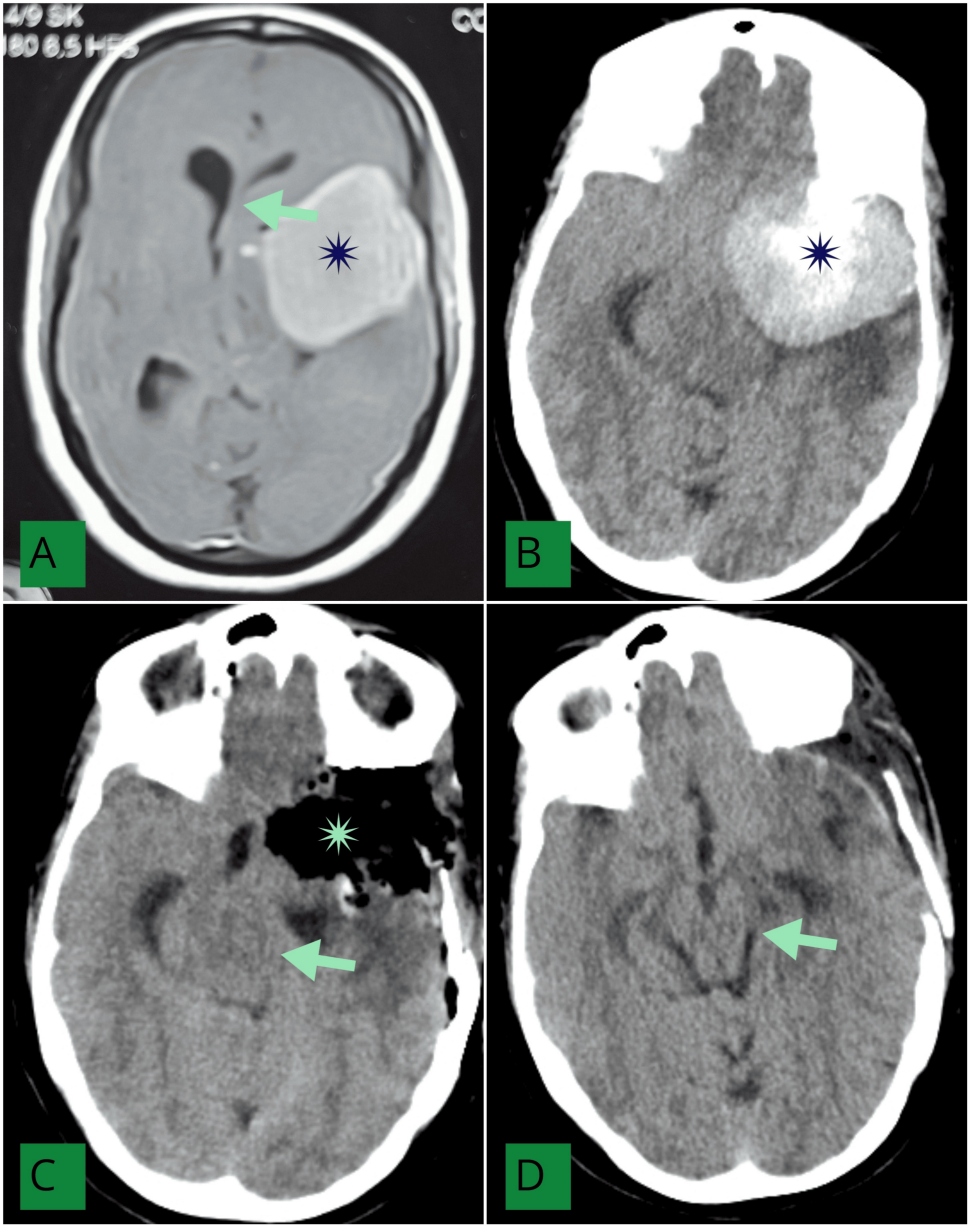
(A) Post-contrast MRI brain (axial view) showing an extra-axial, homogeneously, and densely enhancing lesion in the left frontotemporal region (asterisk) with associated midline shift (green arrow). (B) Preoperative non-contrast CT scan (axial view) demonstrating a hyperdense lesion arising from the left sphenoid wing, displacing the brainstem contralaterally (asterisk). (C) Immediate postoperative non-contrast CT scan (axial view) showing complete resection of the lesion (Simpson grade I) (asterisk). The midline shift persists in the immediate postoperative period (green arrow). (D) One-month postoperative CT scan showing resolution of the midline shift (green arrow). Postoperative CT scan at one-month follow-up demonstrating minor bone-flap malalignment without clinical consequences. The patient had an uneventful recovery. MRI: Magnetic resonance imaging, CT: Computed tomography
